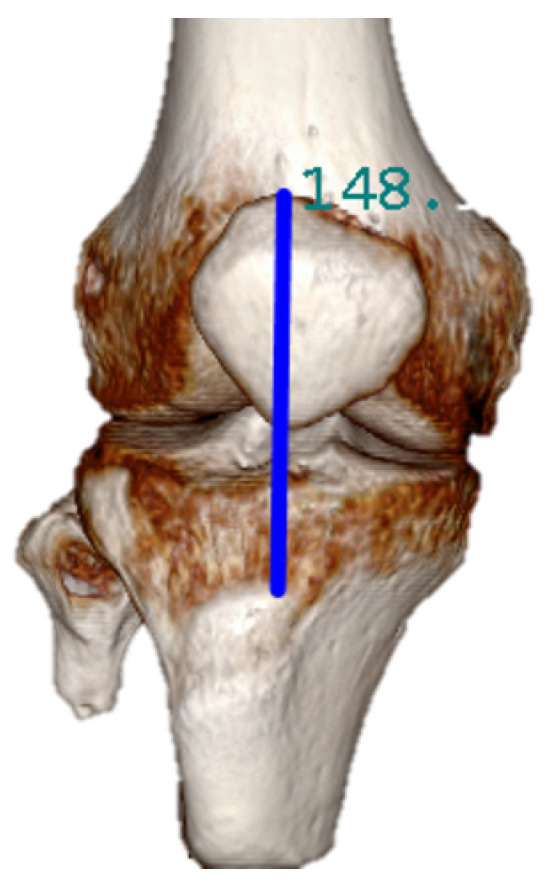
Three-dimensional CT image of the transtibial amputee participant.The measurement to localate the first critical regions of interest (CROI) is taken from the superior point of the patella to the middle point of the tibial crest.
